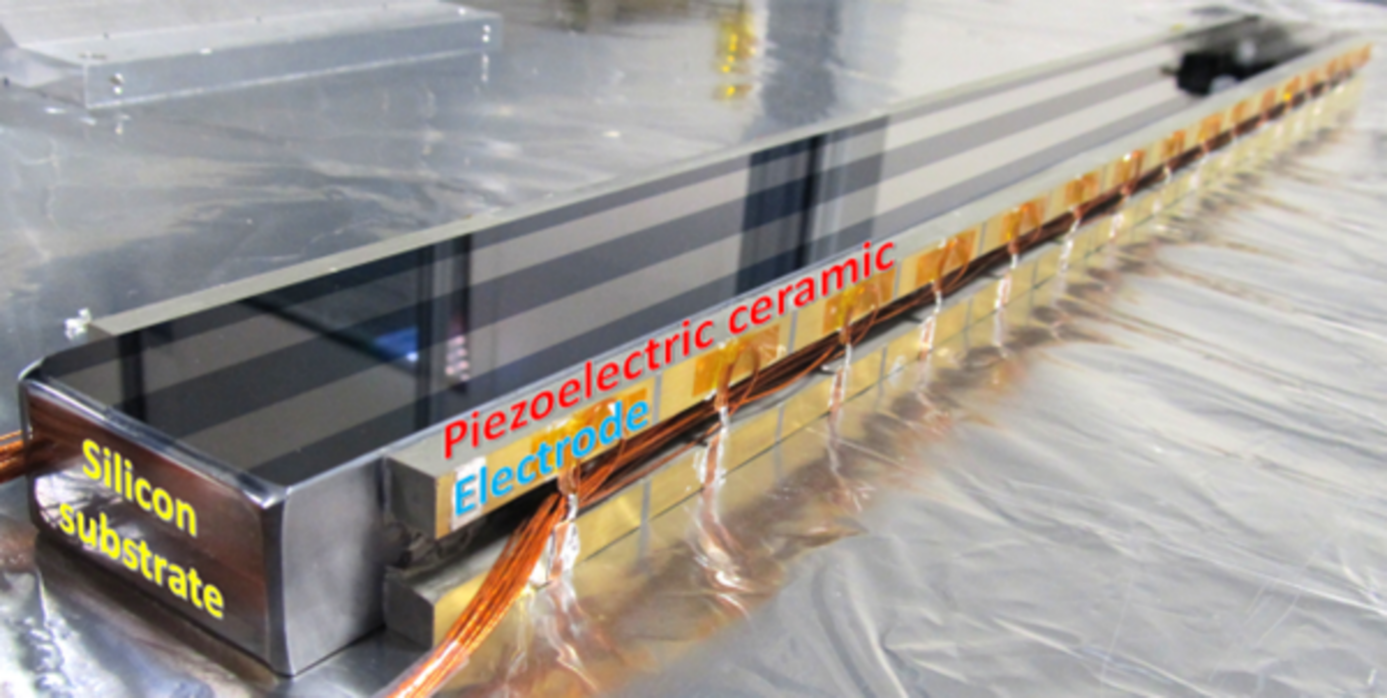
Second-generation bimorph deformable X-ray mirror (Thales-SESO patented), dismounted from its opto-mechanical holder, showing the piezoceramic bars bonded to the side faces of the silicon substrate. Distinct, gold-coloured metallic regions define the actuation region of each piezo electrode.
